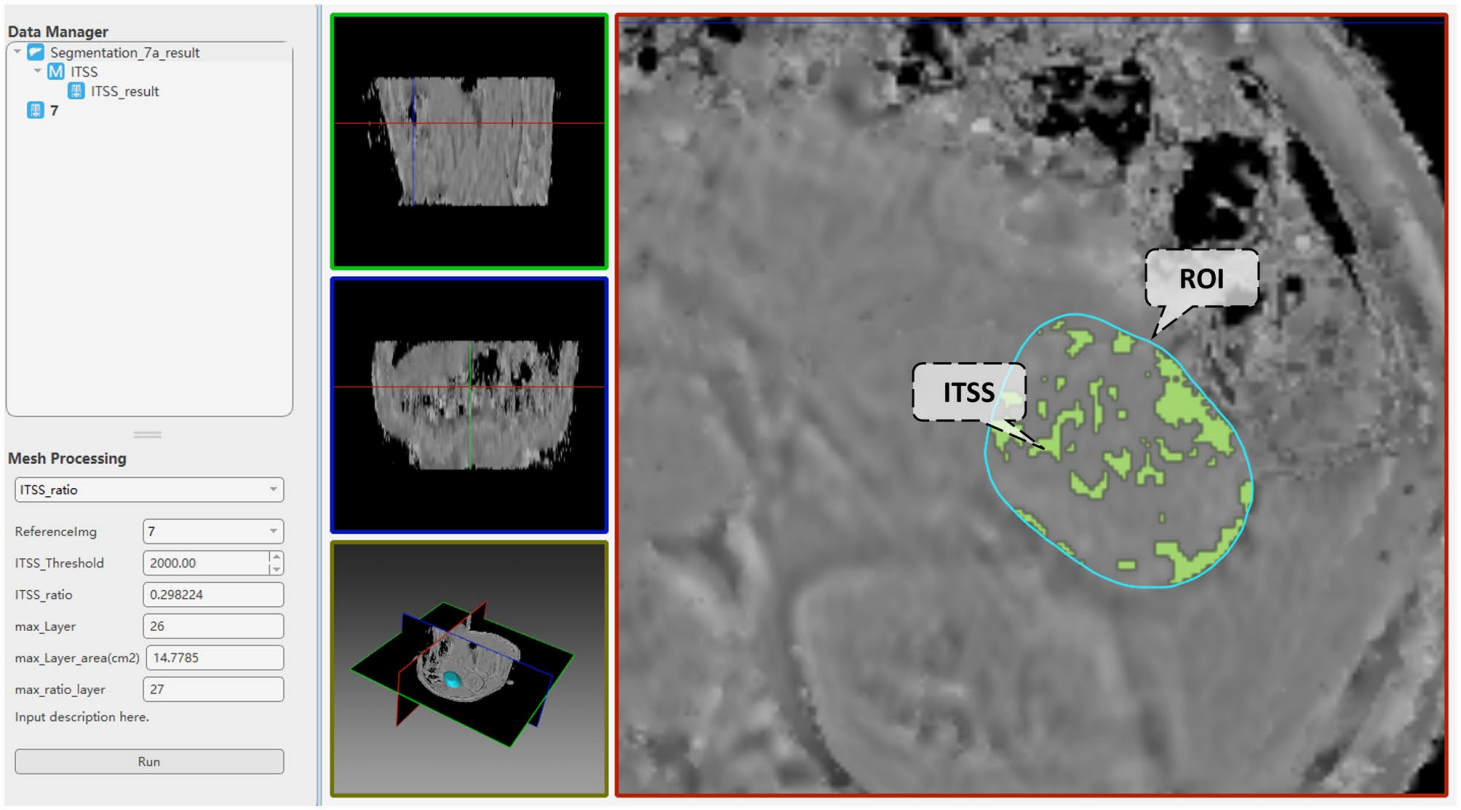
An example of user-defined extension module for ITSS analysis in MR images
